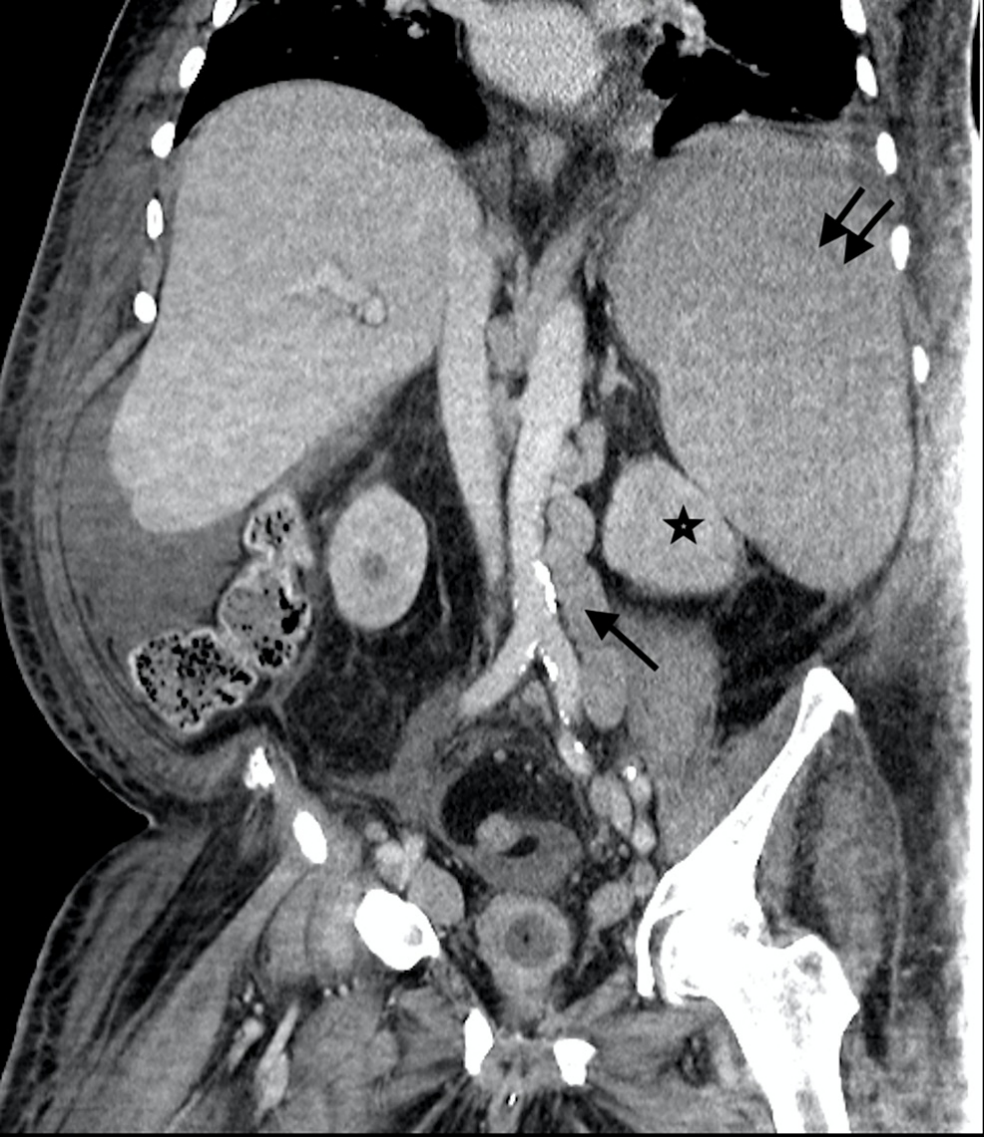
Coronal CT abdomen showing extensive para-aortic lymphadenopathy (arrow), with a large necrotic lymph node (star) and massive splenomegaly (double arrows).
